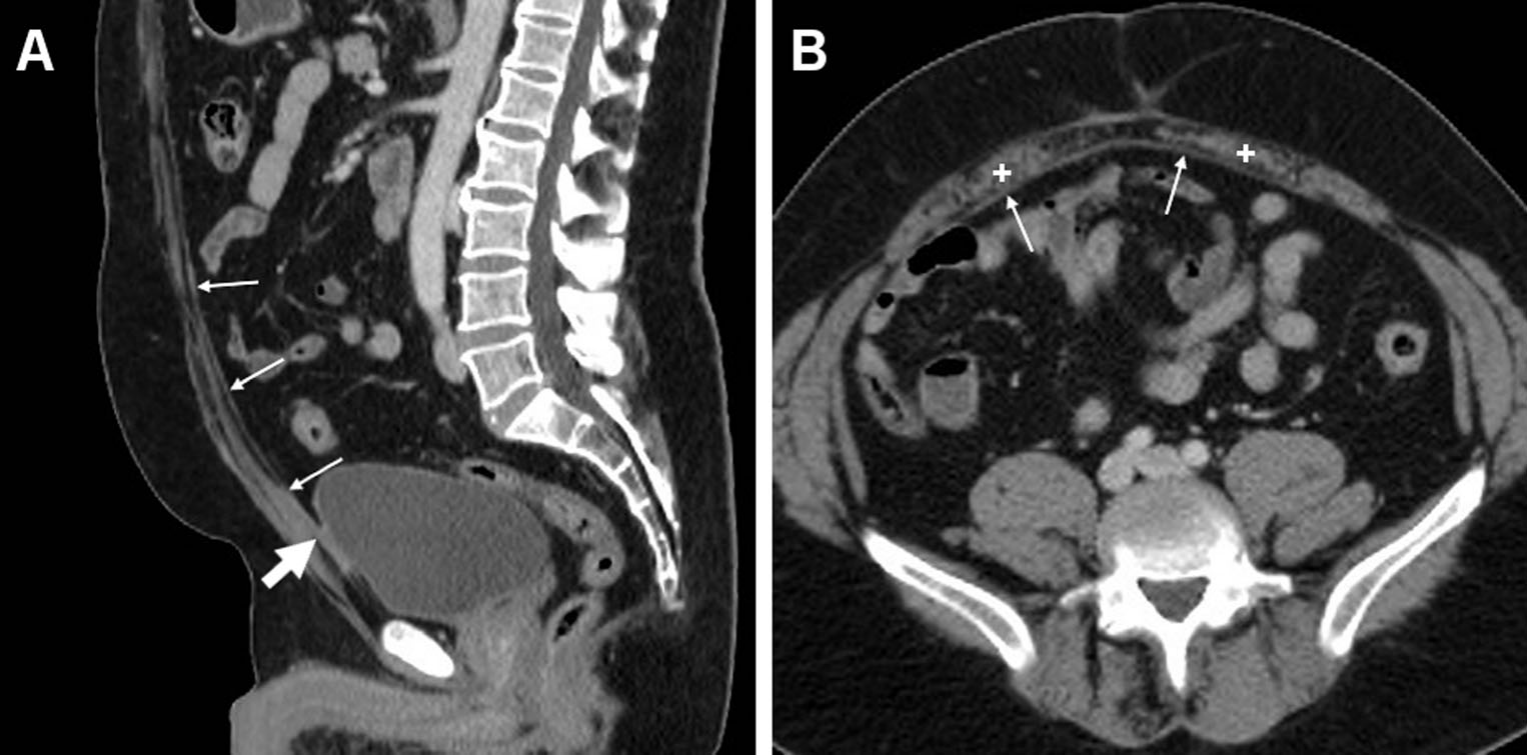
Usual imaging appearance of polypropylene (PP) prosthetic mesh (PM) in a 72-year-old male, two weeks after uncomplicated open repair (Rives-Stoppa technique) of a large ventral incisional hernia. On sagittal (a) and axial (b) post-contrast CT images, a thin structure (thin arrows) with soft-tissue attenuation is identified at the deep aspect of the atrophic rectus abdominis muscles (+), which corresponds to the PP PM interposed between the parietal peritoneum and muscle sheath. Asymptomatic adhesion of the caudalmost portion of the PM to the urinary bladder (thick arrow in A) was incidentally noted
